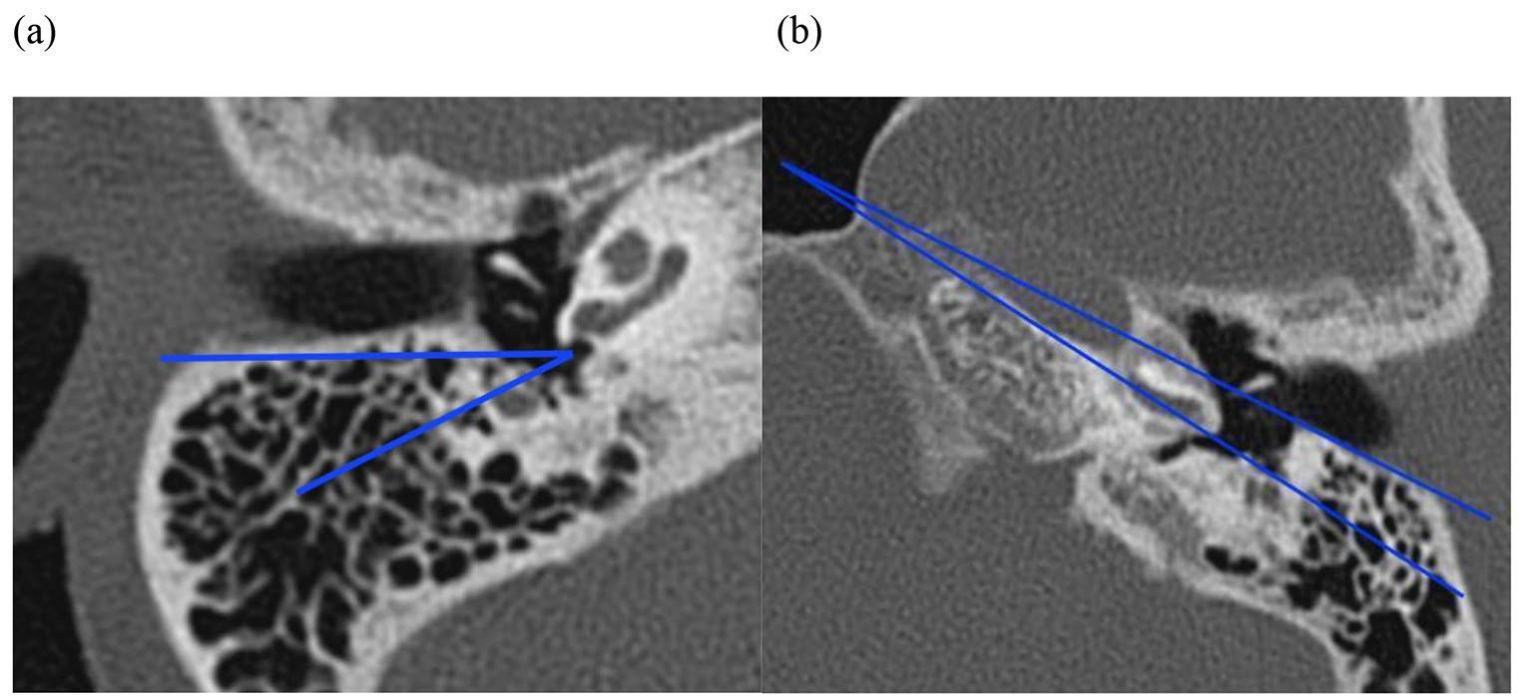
(a) Computed tomography scan of the mastoid, right ear. The measurement described by Rajati et al. [18] is indicated by the angle between the two lines. Code: Rajati_Angle. (b) Computed tomography of the mastoid, left ear, axial plane. The measurement described by Kashio et al. [20] is indicated by the angle between the two lines. Code: Kashio_Angle
